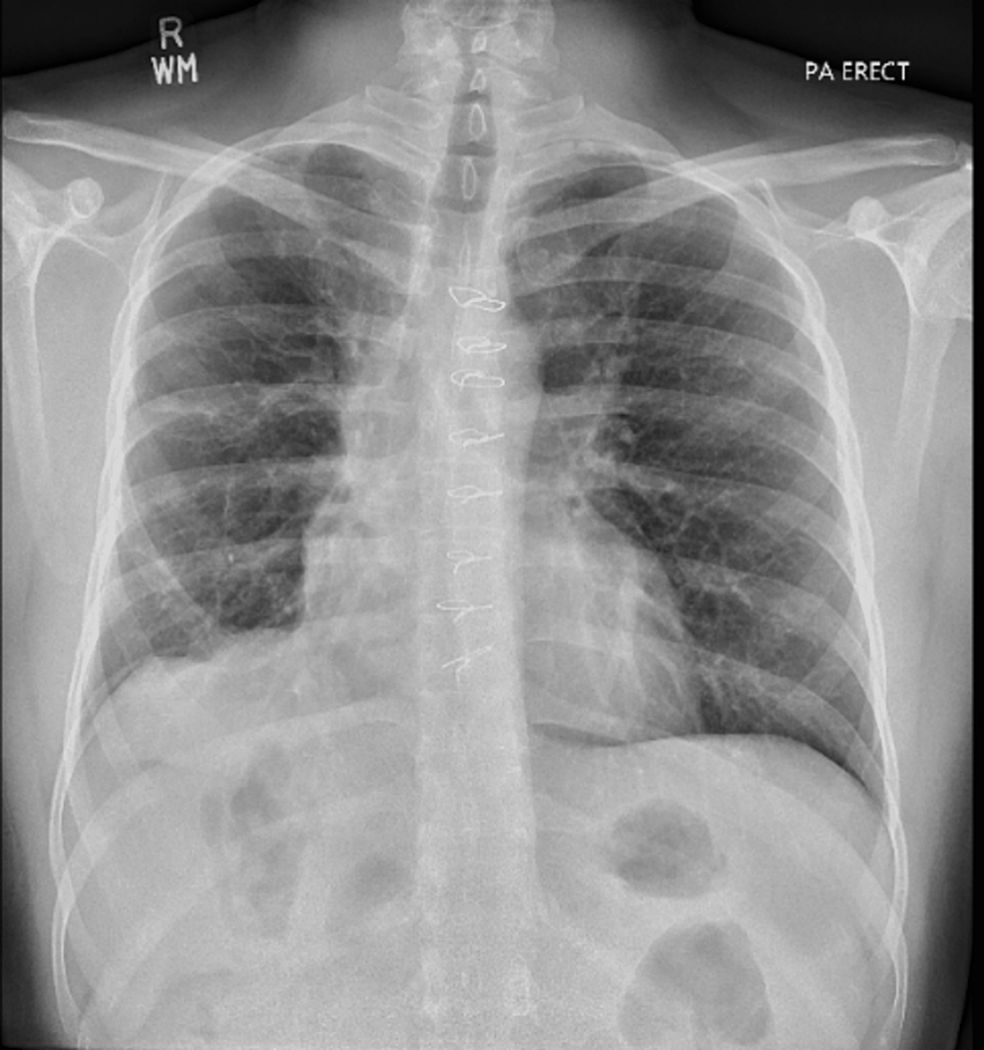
Post-operative follow-up chest x-ray (CXR) This CXR is three years after surgery and showed no disease recurrence
